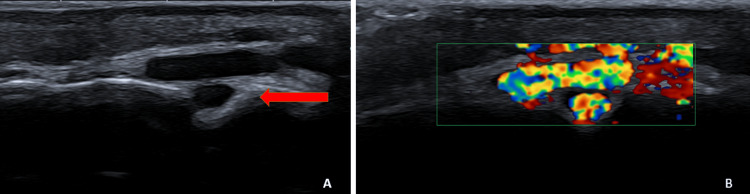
Ultrasound imaging of the scalp mass (A) B-mode ultrasound image showing a vascular mass in the scalp. A possible intracranial extension through a bony defect is suggested (red arrow). (B) Color Doppler ultrasound demonstrating significant vascular flow within the lesion
